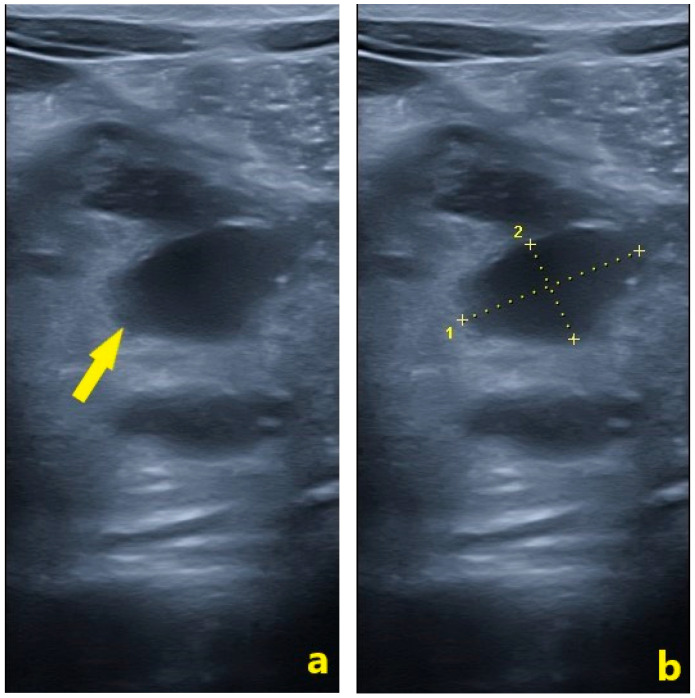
Abdominal ultrasound performed on day 6 post-injury. (a) The solid yellow arrow indicates a well-circumscribed anechoic peripancreatic fluid collection anterior to the pancreatic head, consistent with an evolving APFC. (b) Calipers indicate the collection’s dimensions: 21 × 12 mm (TS × AP).
